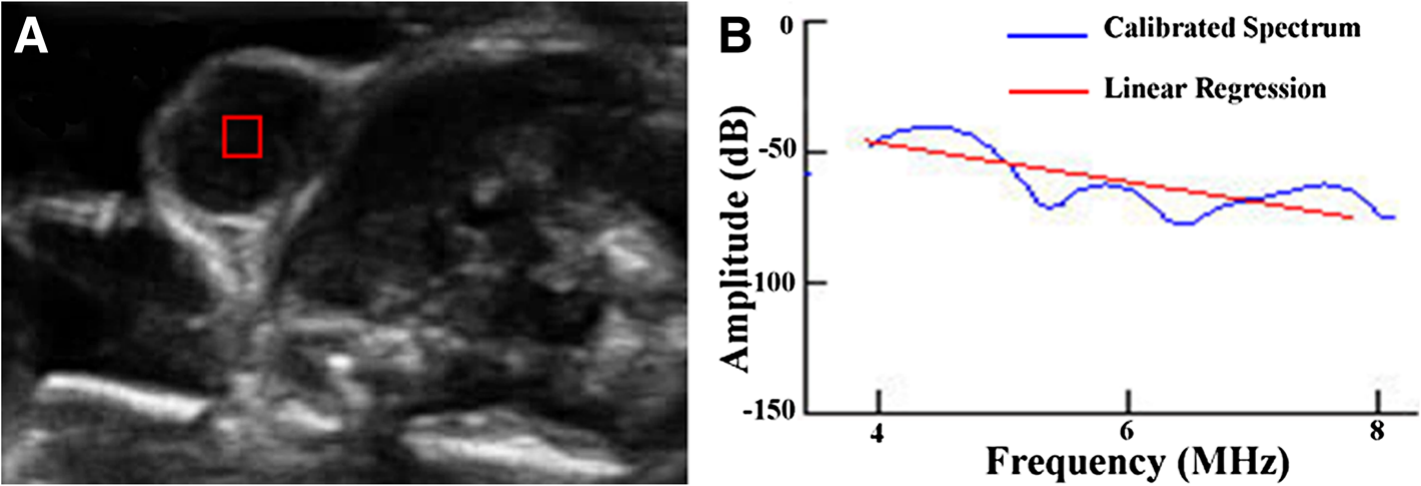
Ultrasound image and power spectrum. (A) Ultrasound image of the MCF7 tumor with the ROI located in the subcutaneous tumor tissue and (B) the corresponding calibrated power spectrum (blue curve) with linear regression (red line).
